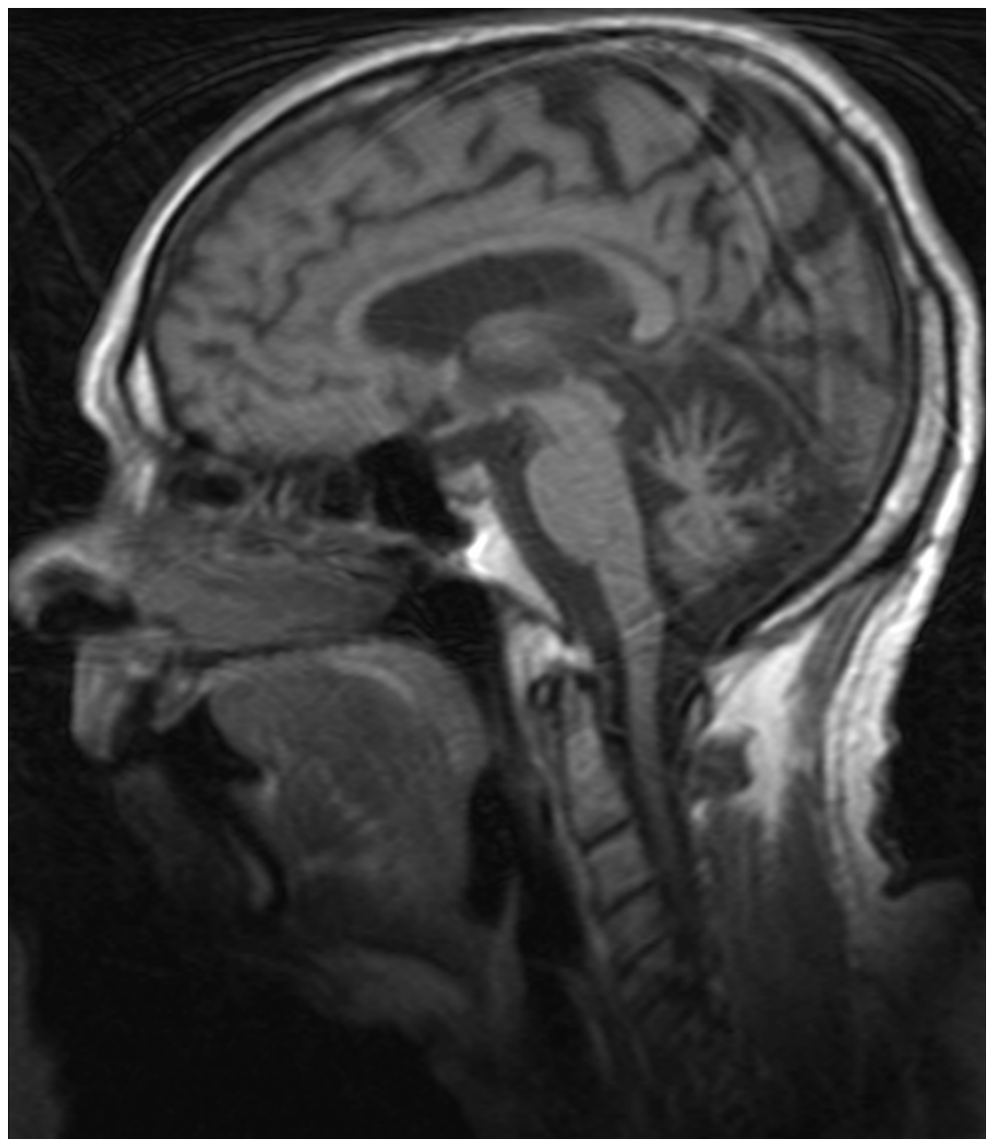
Brain MRI of patient AIV-5 showing cerebellar atrophy.
